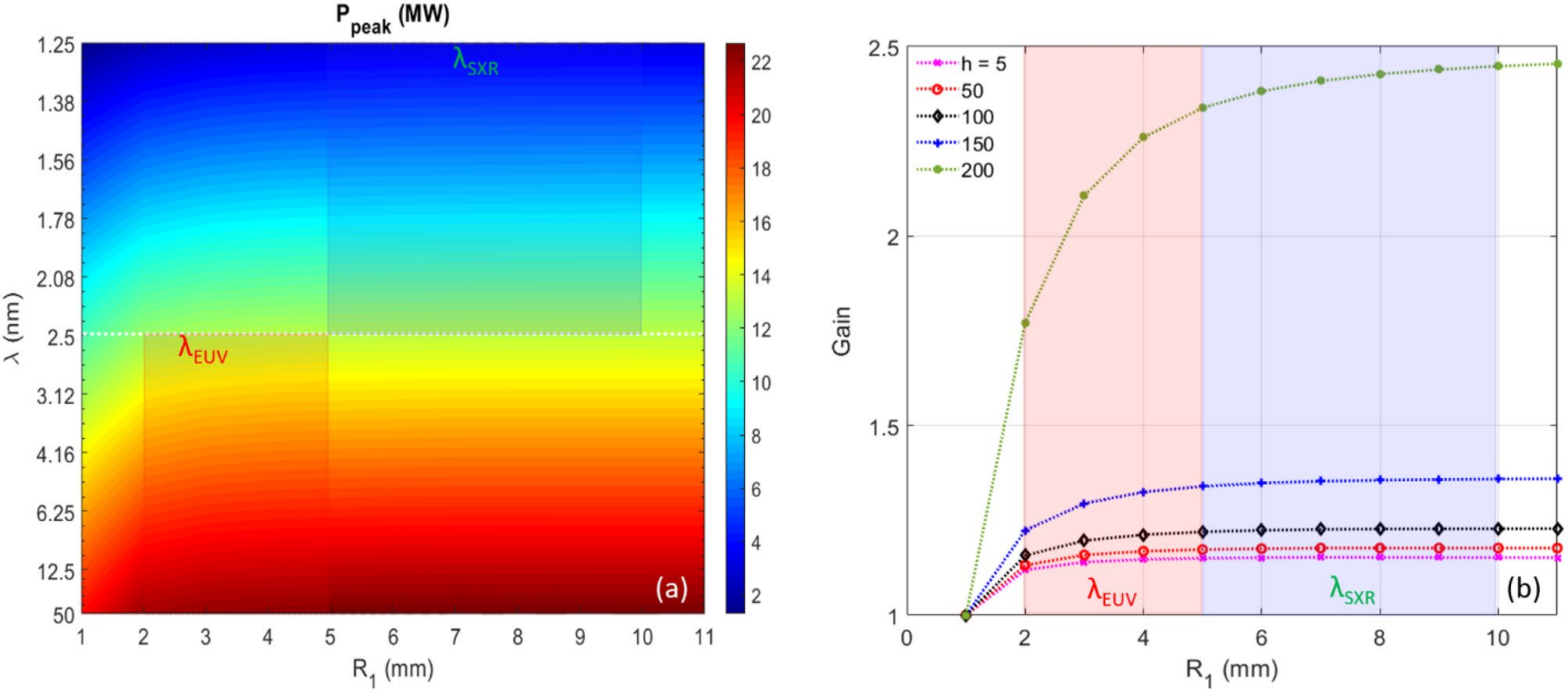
For peak current of 300 A, (a) Peak power as functions of $$\lambda$$ (y axis) and $$R_{1}$$ (x axis) is shown as the contour plot. The EUV and soft X-ray beamlines are labelled as $$\lambda_{EUV}$$ and $$\lambda_{SXR}$$, respectively. (b) Gain as a function of $$R_{1}$$ is plotted for harmonics 5 (magenta), 50 (red), 100 (black), 150 (blue), and 200 (green), respectively. The EUV and soft X-ray beamlines are highlighted as pink and light blue, respectively.
